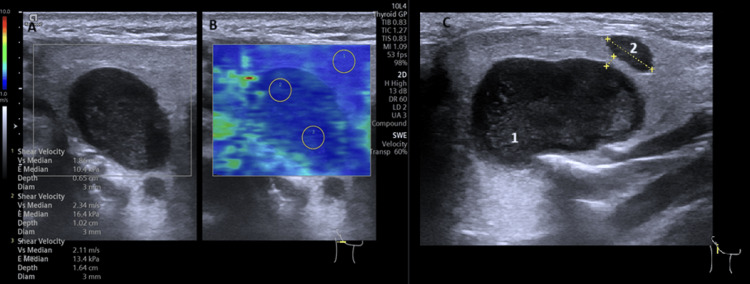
(A) B-mode ultrasound image showing a hypoechoic lesion with regular borders in the superficial lobe of the right parotid gland. (B) VTIQ of the same tumor indicating low stiffness. (C) Intraparotid tumor (1) and a secondary adjacent smaller hypoechoic nodule (2). VTIQ: Virtual Touch Imaging Quantification Elastography.
